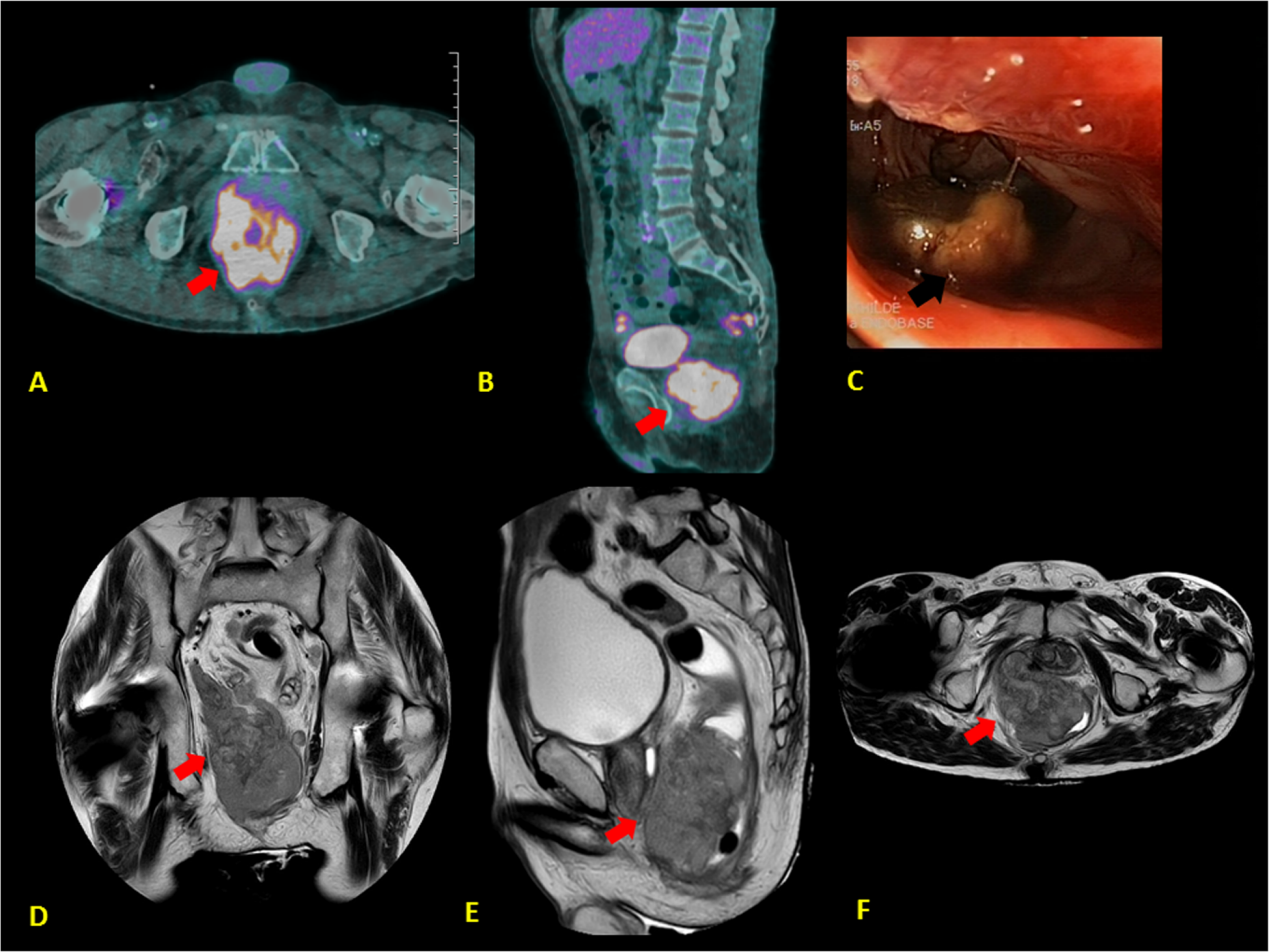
Diagnosis of a primary, anorectal small cell tumor. a-b Voluminous, hypermetabolic anorectal tumor lesion (PET-scan: axial and sagittal section; red arrows) diagnosed after 6 cycles of chemotherapy. c Tumor lesion of the right wall of the anorectal region (Colonoscopy; black arrows). d-f Voluminous tumor of the right rectal wall of 62 × 84 × 162 mm of diameter, infiltrating the prostate, the right seminal vesicle, the internal anal sphincter, the levator ani and the puborectalis muscle (pelvic MRI: coronal, sagittal and axial section; red arrows)
